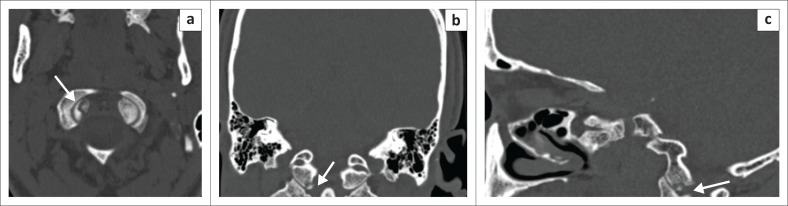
(a) Axial, (b) coronal and (c) sagittal computed tomography bone window with a right occipital condylar fracture (arrows).
